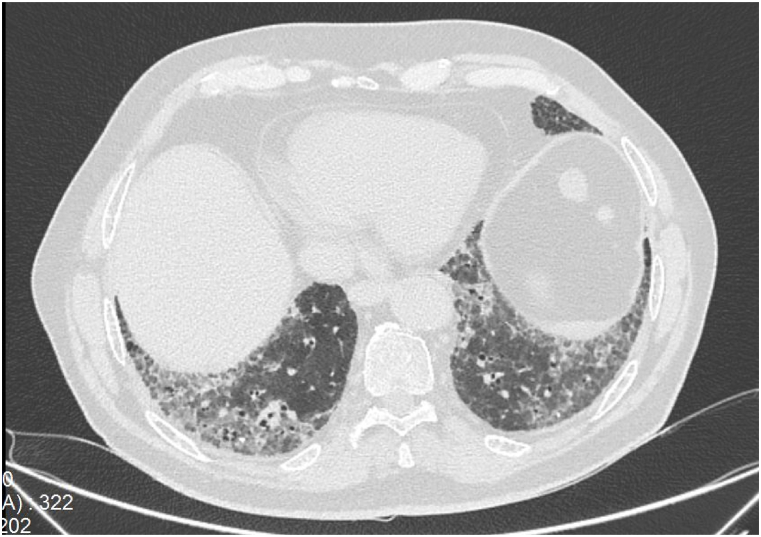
High-resolution computed tomography (HRCT) scan of the chest at the level of the lower lung fields, showing signs of pulmonary fibrosis, including bilateral interstitial infiltrates with reticular patterns and areas of ground-glass opacities.
